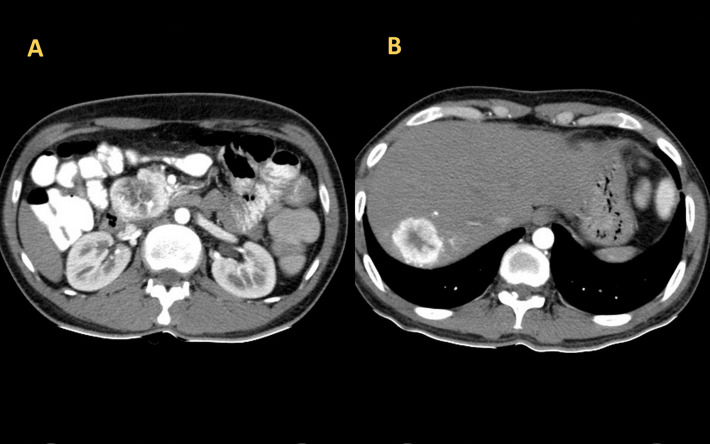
Axial section image of CT scan showing heterogeneously enhancing mass lesion in the head of pancreas (A) and right lobe of liver (B)
